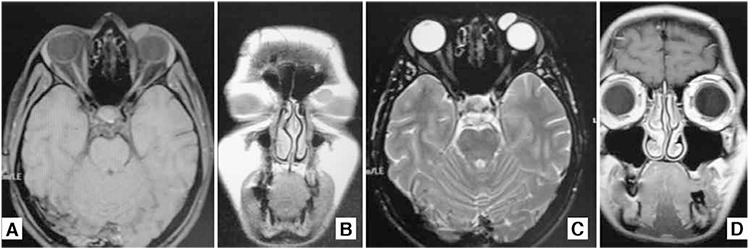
Magnetic resonance imaging of the mass: A Mild hyperintensity on T1-weighted horizontal section; B. Marked hyperintensity on T2-weighted horizontal section; C and D. In coronal section the mass is visible superomedially adjacent to the left globe
